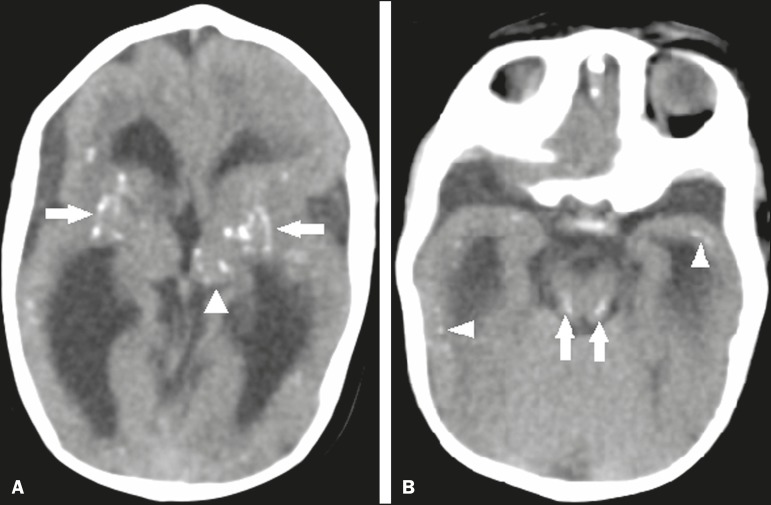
A: A 5-month-old patient. Axial CT scan, without contrast, showing foci
